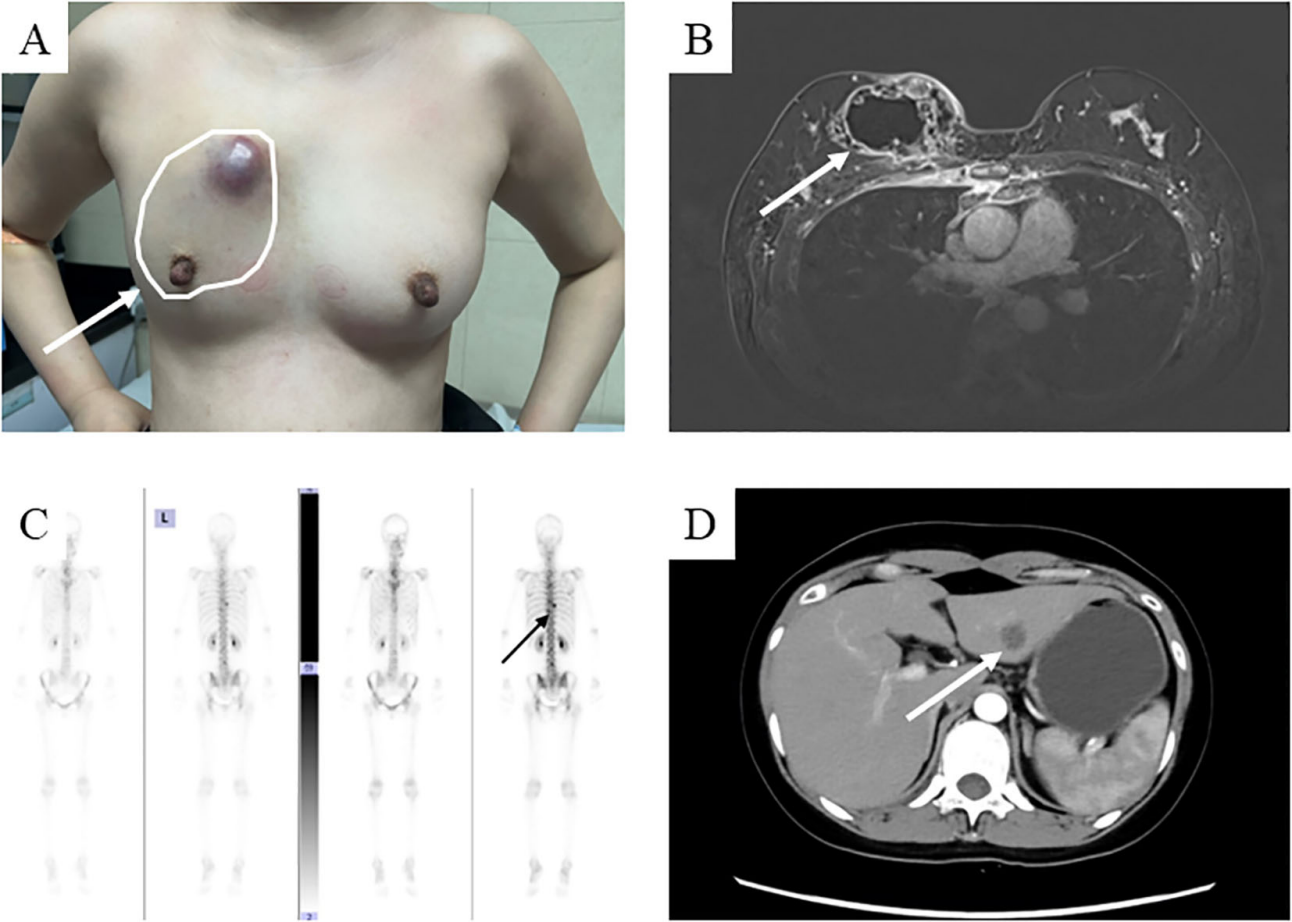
The condition of the patient at the time of their initial visit. (A) A hard mass measuring 10x8 cm with unclear borders and poor mobility is present in the right breast, accompanied by localized skin protrusion and redness; (B) The breast mass is shown on the enhanced magnetic resonance imaging scan. (C) Enhanced Computed tomography images scan shows liver metastasis; (D) Whole Body Scan suggests metastatic lesions at the 5th, 8th, and 9th thoracic vertebrae.
